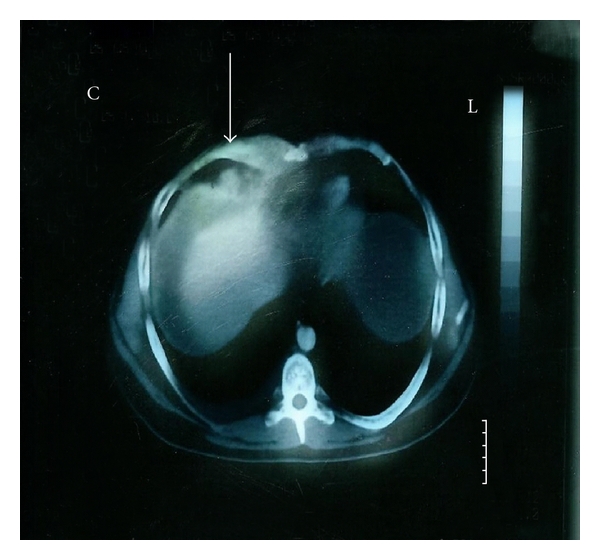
Axial enhanced CT-image of the lower part of the chest (mediastinal window) shows small pleural effusion and an epiphrenic node at the right. Ascites and thickness of the right crus are also noticed.
